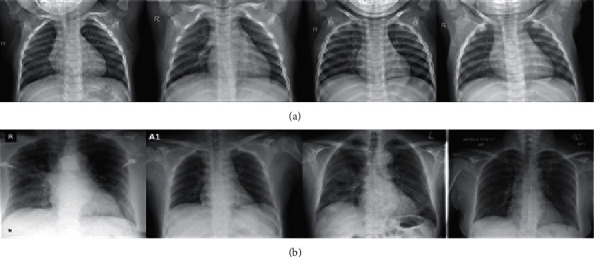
(a) COVID-19 chest X-ray; (b) normal chest X-ray [24].
